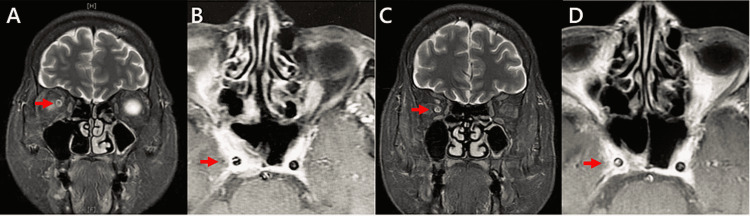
Magnetic resonance imaging. (a) A coronal view reveals a contrast-enhanced right optic nerve sheath of the first attack (arrow). (b) An axial view of T1-weighted MRI shows contrast-enhanced cavernous sinusitis of the attack (arrow). (c) A coronal view of third attack revealing a residual contrast effect around the right optic nerve (arrow). (d) An axial view of T1-weighted MRI showing a reduced contrast-enhanced of cavernous sinusitis of the third attack (arrow).
